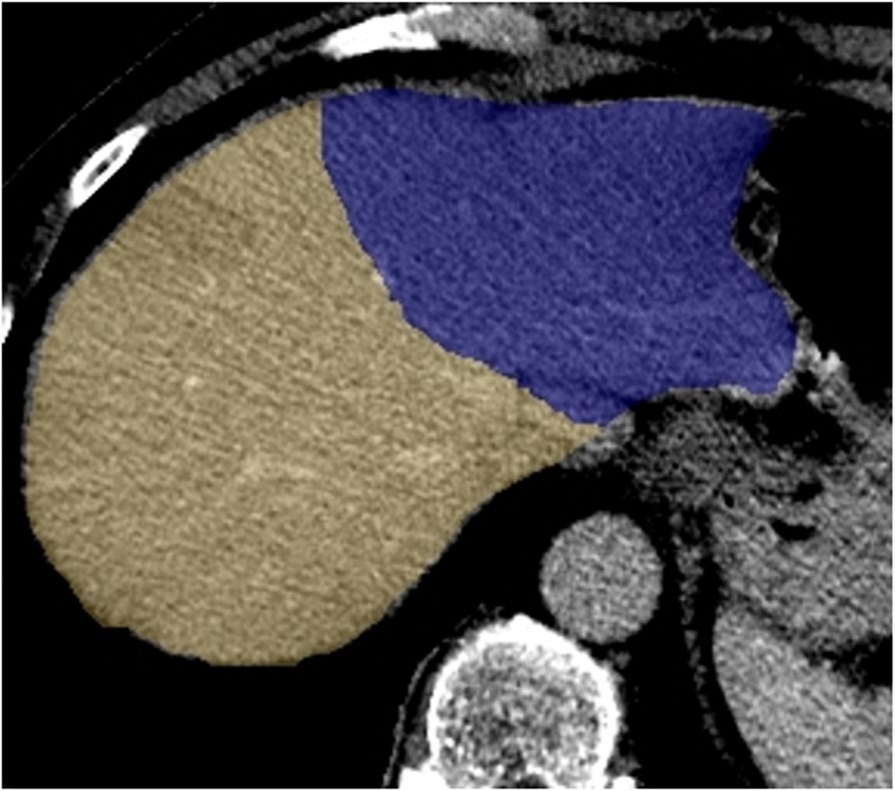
Semi-automated segmentation of contrast enhanced CT of the liver into left and right lobes
